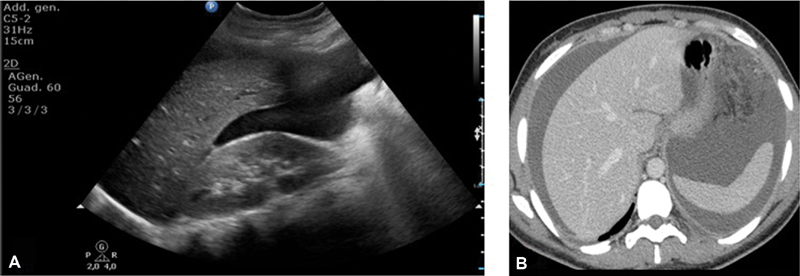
Copious ascites on transabdominal ultrasound (A) and CECT (B).
